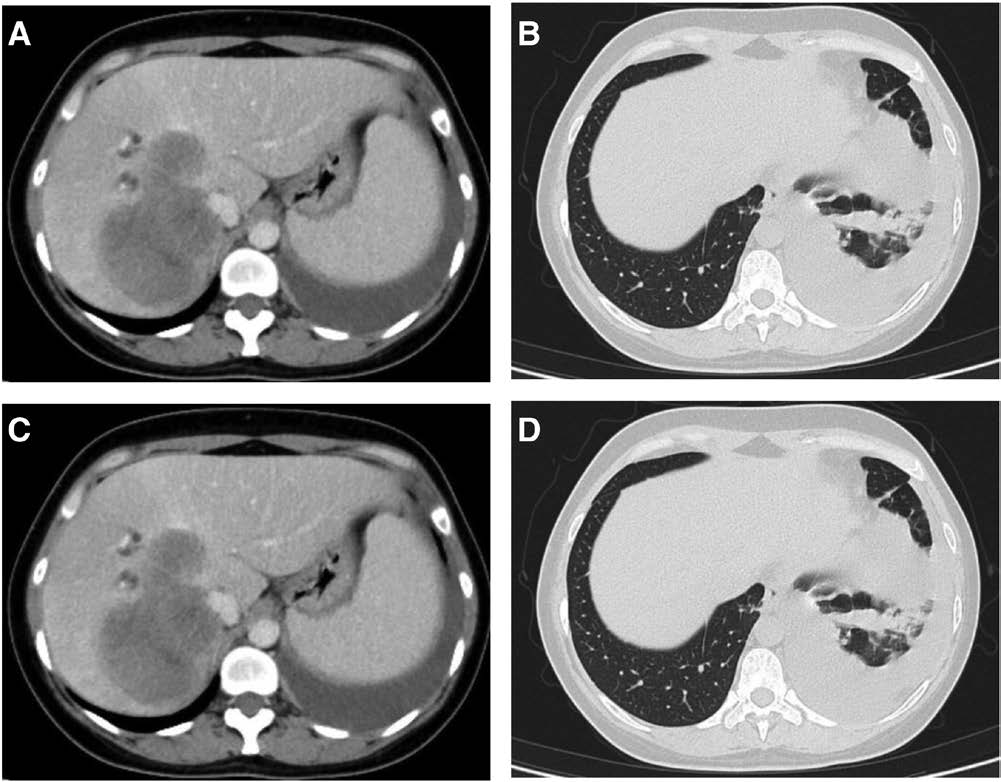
CT scans for (A and B) June 2021 and (C and D) August 2021. CT = computed tomography.
